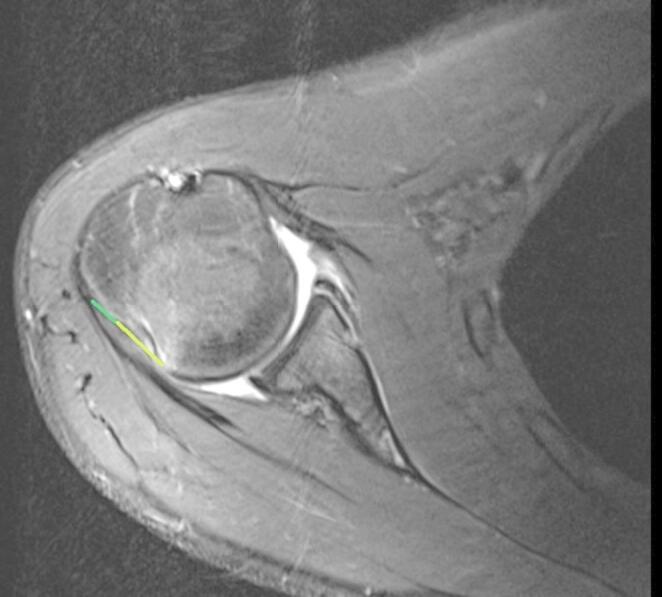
Measurement of the Hill-Sachs Index using MRI as an example. First, measurement of the Hill-Sachs lesion (light green line) and measurement of the bony bridge (green line). Then addition of the two measured distances
